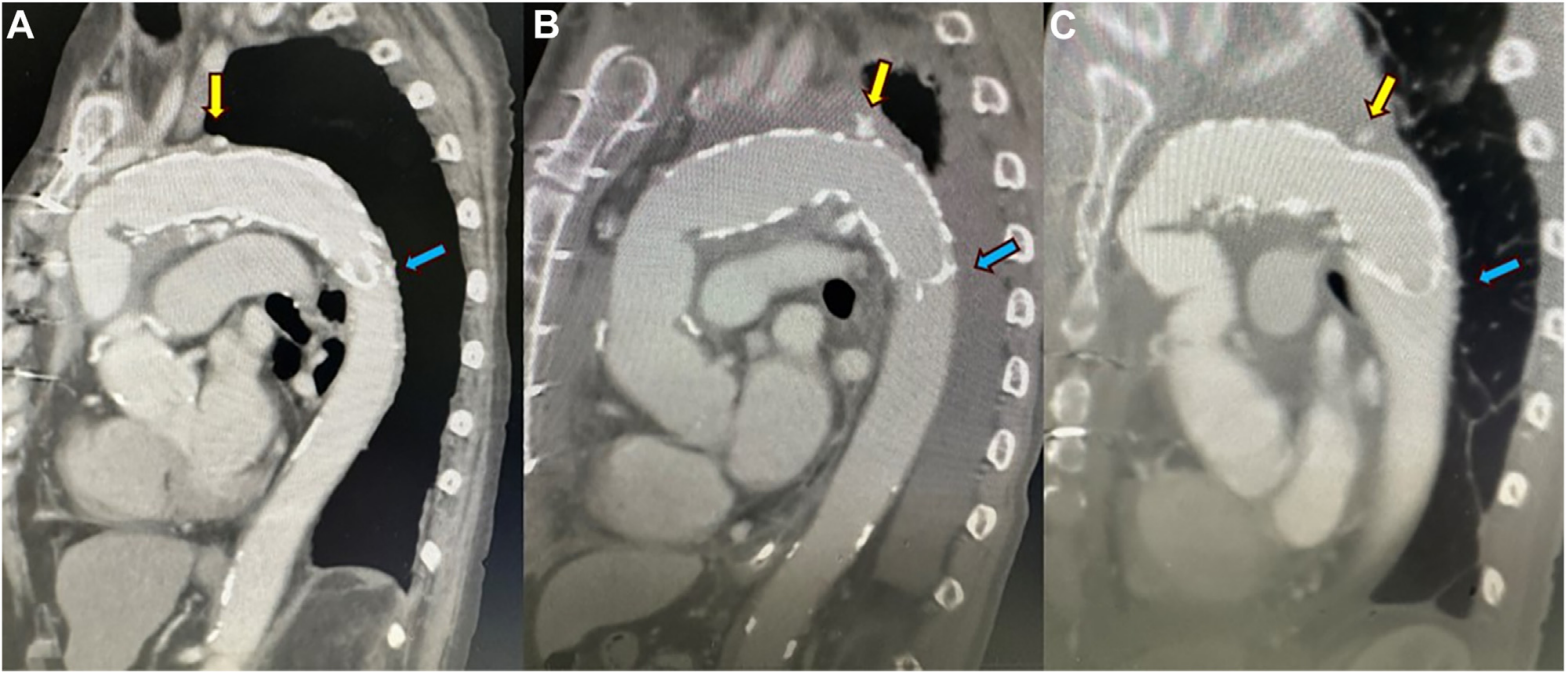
Postoperative computed tomography image of each patient. (A, B) Patients 1 and 2 with infectious aortic aneurysms. (C) Patient 3 with aortic coarctation. Yellow arrows indicate the distal anastomosis line. Blue arrows indicate the distal edge of the frozen elephant trunk.
